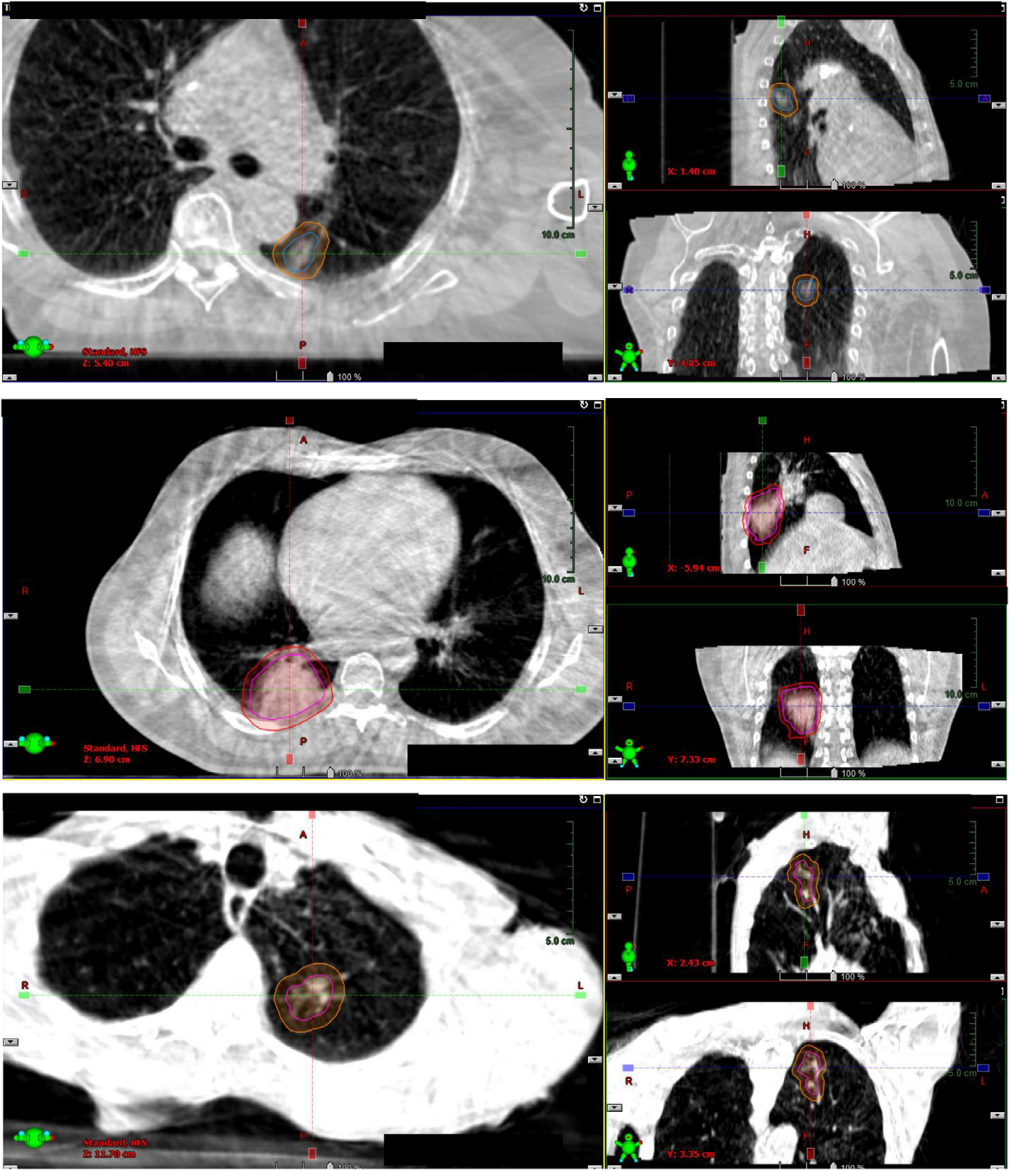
SKIM CBCT alignment with the iGTV and PTV displayed based on the CBCT alignment for patient 8 (LLL target), patient 1, and patient 6. These cases represent a moderate, high, and low degree of tumor motion, respectively.
